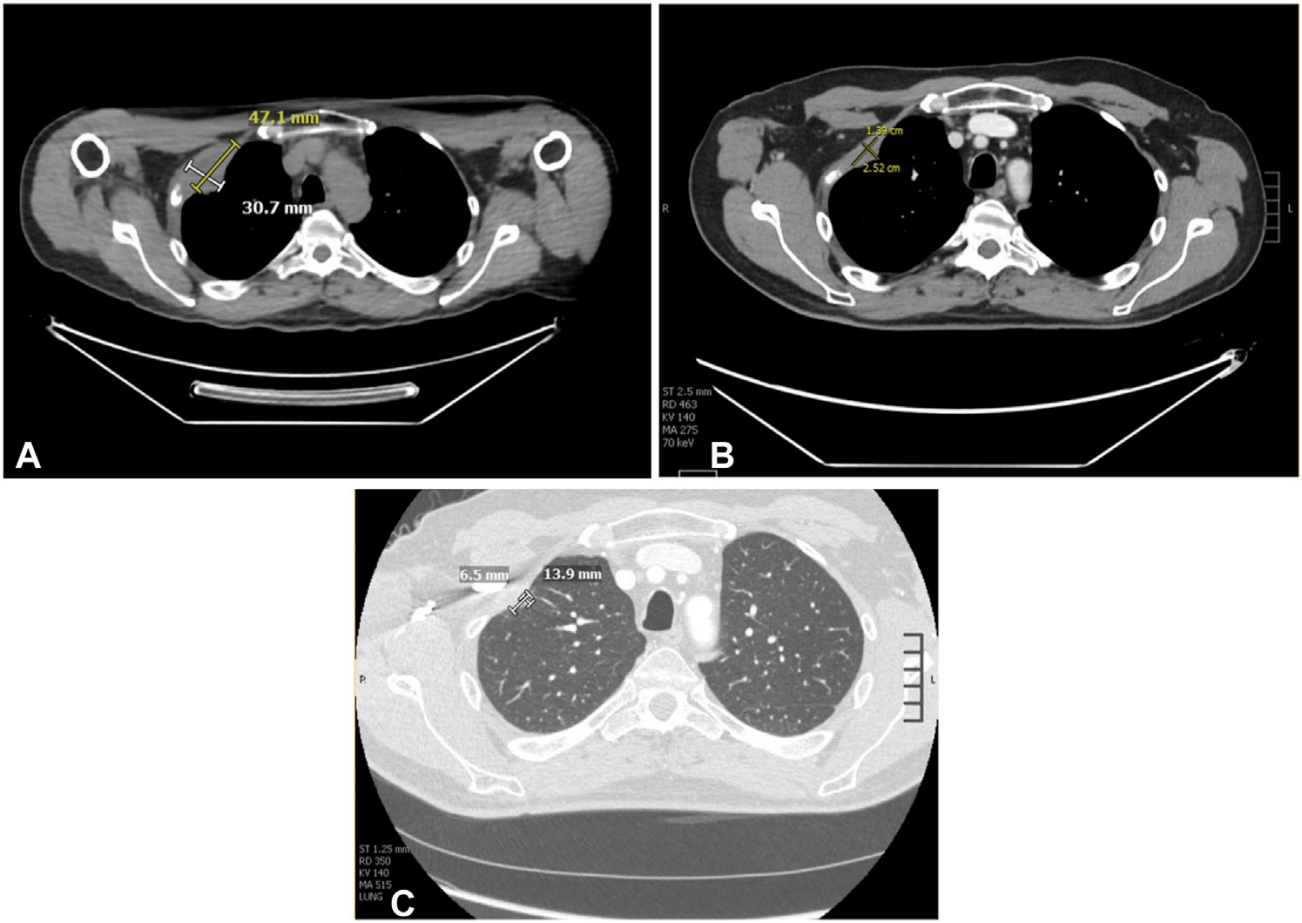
Radiographic presentation of right upper chest wall mass pretreatment and post-treatment with pembrolizumab (initiated in September 2022) in a case of metastatic cutaneous squamous cell carcinoma. A, Represents imaging 1 month prior to treatment with pembrolizumab, (B) represents imaging 6 months into treatment with pembrolizumab, and (C) represents imaging 18 months into treatment with pembrolizumab. A, Radiographic presentation of right upper chest wall mass measuring 4.7 × 3.0 cm in August 2022. B, Radiographic presentation of right upper chest wall mass measuring 1.4 × 2.5 cm in January 2023. C, Radiographic presentation of right upper chest wall mass measuring 0.65 × 1.39 cm in January 2024.
